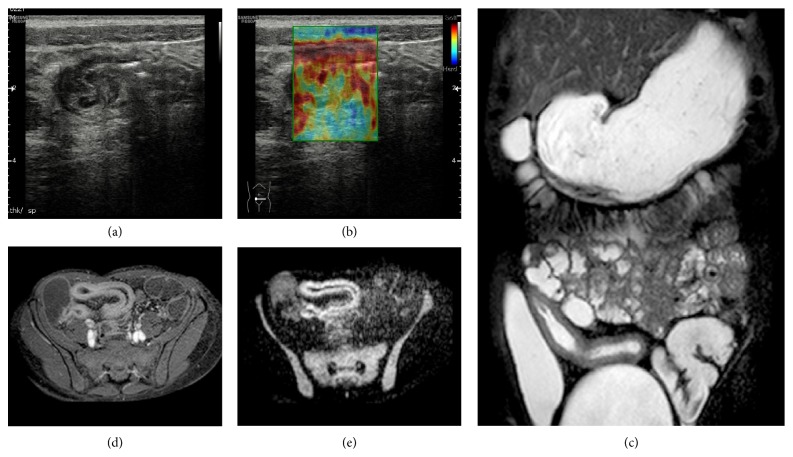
Longitudinal US-scan of the last ileal loop in a 33-year-old male shows wall thickening with prominence of submucosa and hyperechoic appearance of perivisceral fat. The gray scale (a) findings and US-SE (b) aspect performed at this level are suggestive for active inflammation pattern. The MR study ((c), (d), and (e)) confirmed these suspicions: T2 weighted coronal view revealed hyperintensity of the bowel wall, T1 weighted axial postcontrast showed stratification of the bowel wall, DWI with 800 *b* value showed hyperintensity of the bowel wall, and an aspect of the last ileal loop is very similar with US findings.
